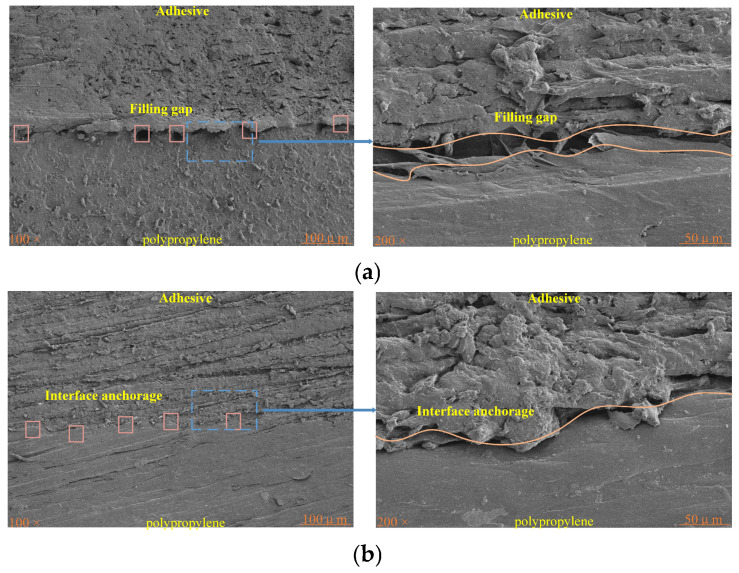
Cross-sectional view of the (a) traditional method and (b) plasma–ultrasonic method.
